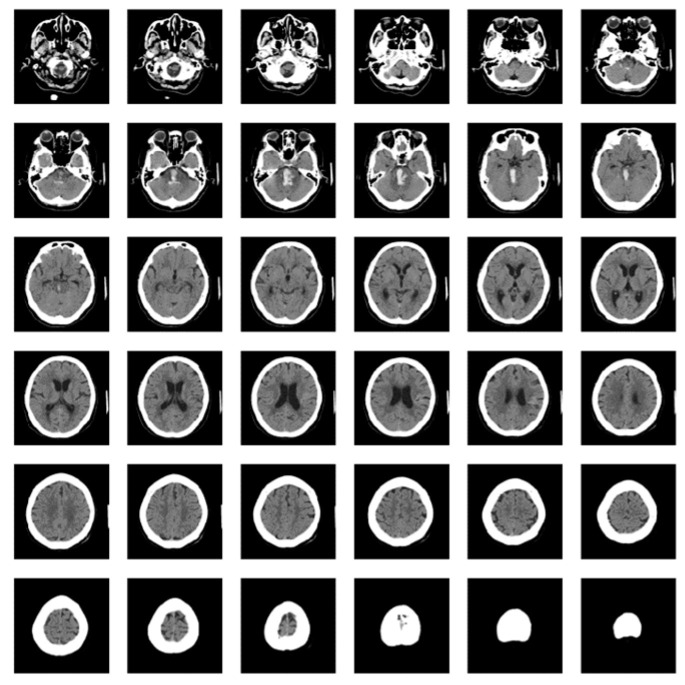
Images of one brain computed tomography dataset.
